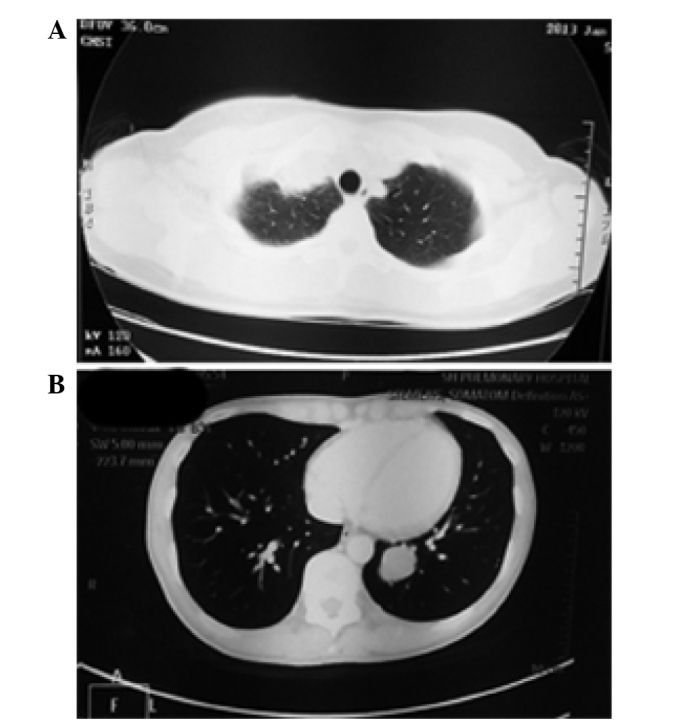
(A) Case 3: A 46-year-old female with no obvious symptoms. Irregular clumps were found below the pleura of the right upper lung on a chest CT during a physical examination. (B) Case 4: A 30-year-old female with no obvious symptoms. Multiple nodules in both lungs were revealed on a chest CT during a physical examination. CT, computed tomography.
